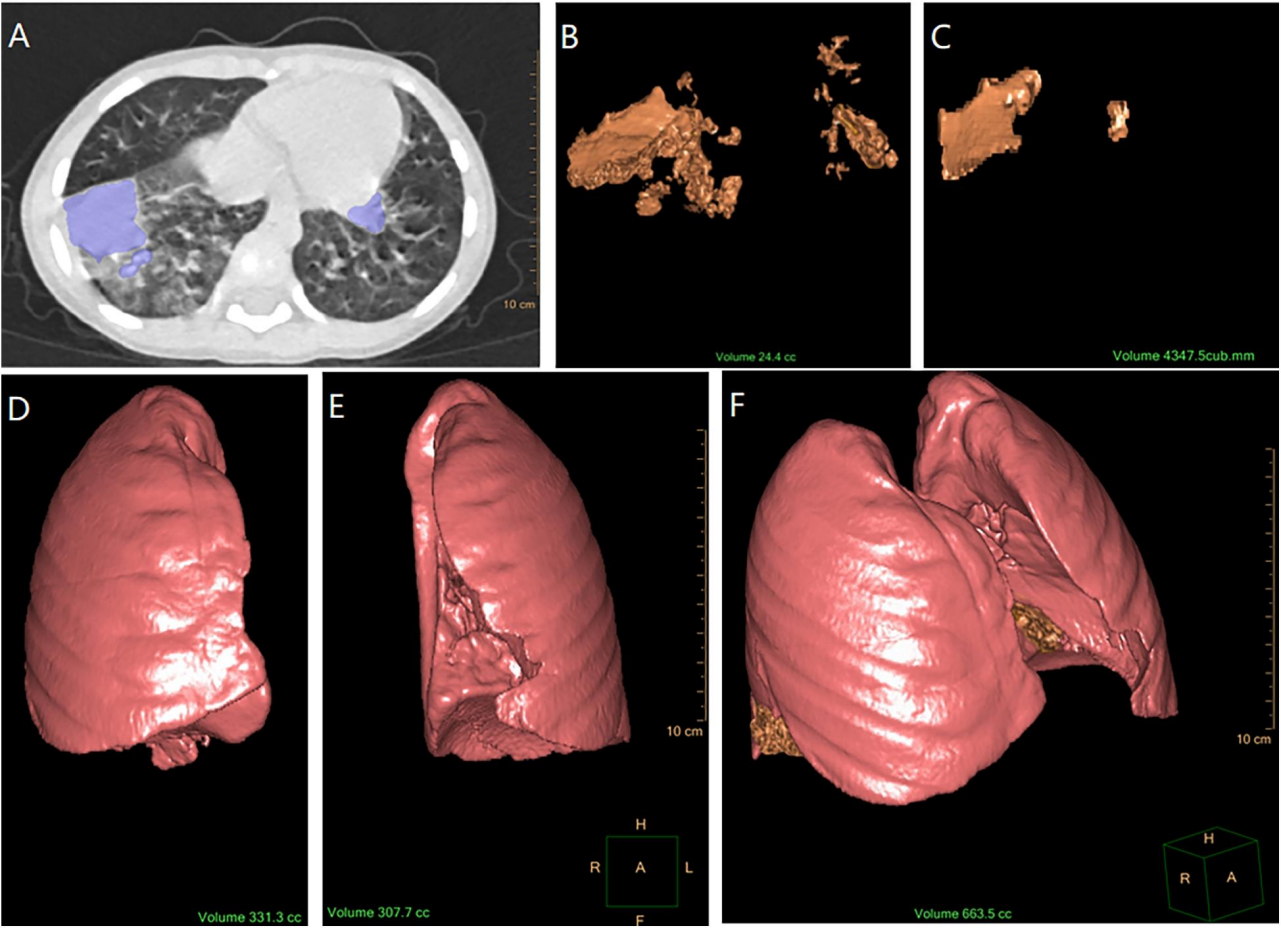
Process of calculating consolidation volume and total lung volume. (A) Marking the consolidation area on CT imaging (by IntelliSpace Portal software). (B) Calculation of initial consolidation volume using 3D reconstruction (the software automatically calculated the volume of the initial pulmonary consolidation delineated and presented it in the form of a 3D reconstruction). (C) Calculation of follow-up consolidation volume using 3D reconstruction (software automatical calculation). (D) Calculation of right lung air density tissue volume using 3D reconstruction (software automatical calculation with the bronchial structures excluded). (E) Calculation of left lung air density tissue volume using 3D reconstruction (software automatical calculation with the bronchial structures excluded). (F) Measurement of the total lung volume [The total lung volume (F) was obtained by adding the initial consolidation volume (B) to the air density volumes of the right and left lungs (D,E), which is F = B + D + E].
